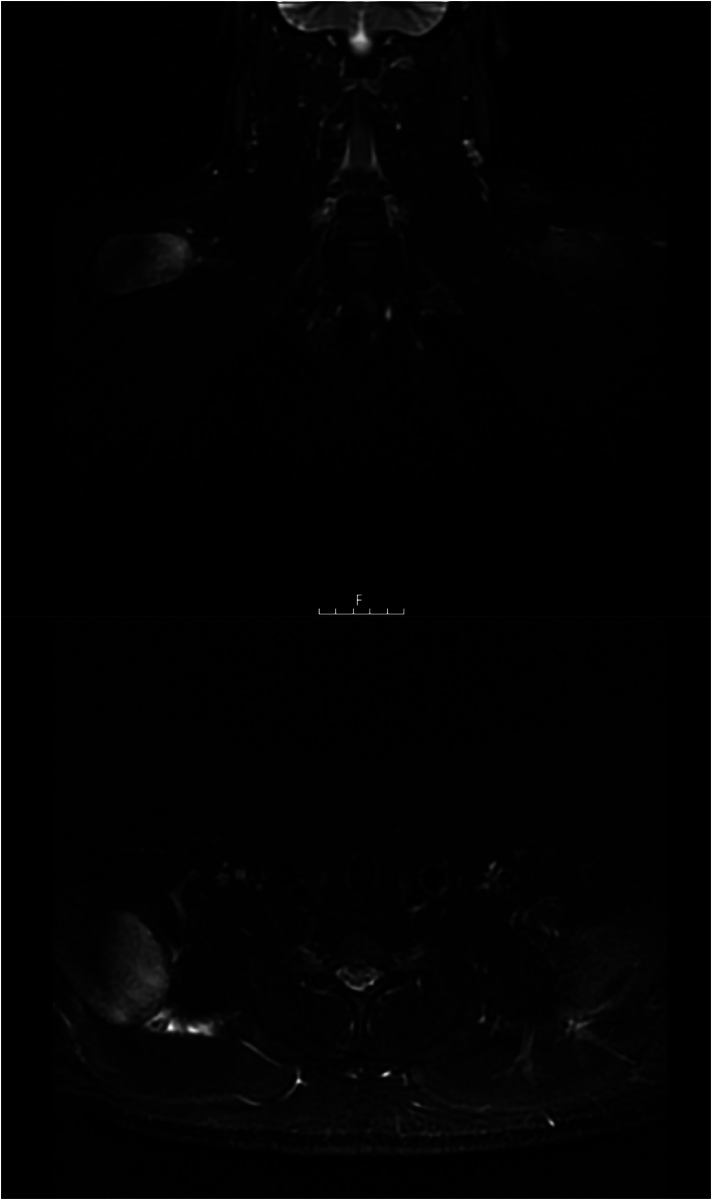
Magnetic resonance imaging of both shoulders demonstrating increased signal and enlargement of the supraspinatus on the right, and mild changes on the left. Short tau inversion recovery (STIR) sequence.
